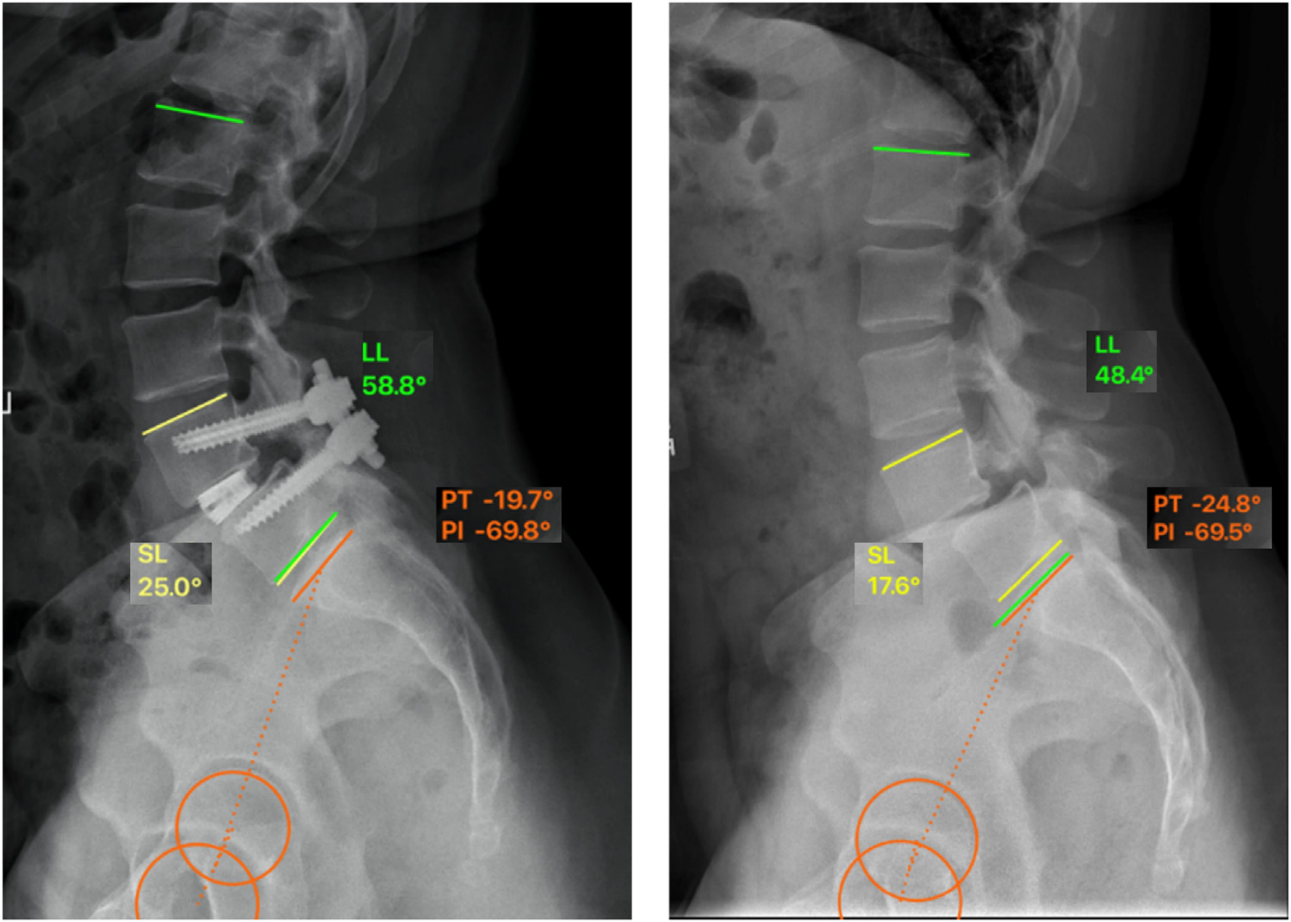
Evaluation of radiographic parameters (LL-lumbar lordosis, SL-segmental lordosis, PT-pelvic tilt, PI-pelvic incidence), DH-disc height was measured between each pair of evaluated operative levels as a distance at anterior and posterior side of the vertebrae.
